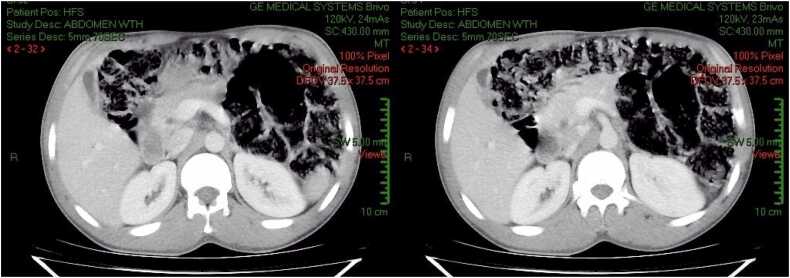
CT Scan: Marked improvement of intra and extra-hepatic biliary duct dilation with slight residual dilation. No visible ductal lesions (Slice 32,34).
